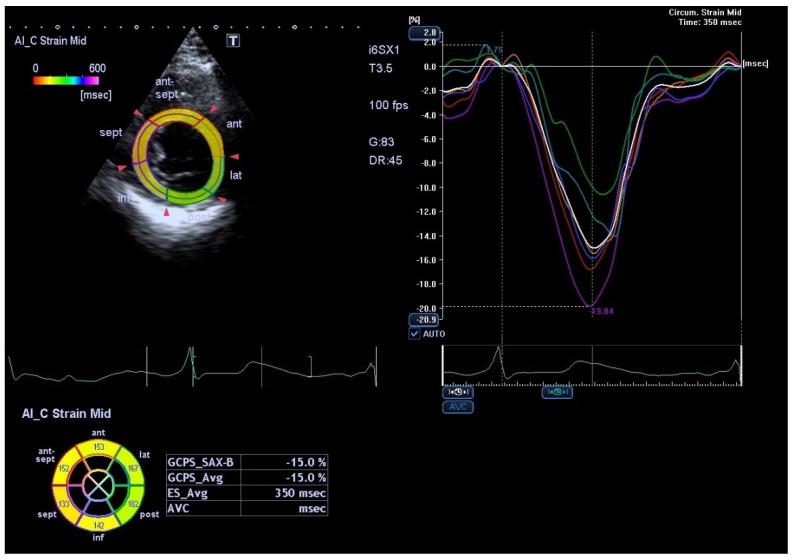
Echocardiography of a healthy pregnant patient showing circumferential left ventricle strain analysis (at the level of the papillary muscles) and speckle tracking analysis. GCPS_SAX-M-Global circumferential strain peak systolic middle left ventricle; GCPS_Avg-Average global circumferential strain; ES_Avg-Average End systolic time. Courtesy of the Fetal Medicine Foundation.
